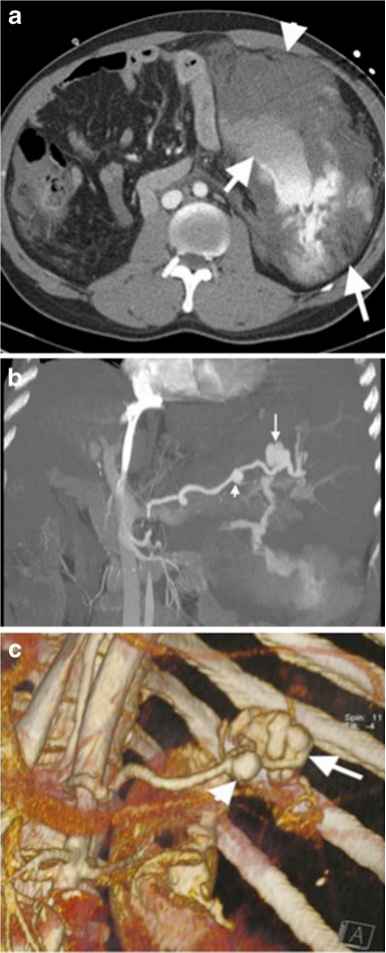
Ruptured splenic artery aneurysm. (a) Axial contrast-enhanced CT of a 57-year-old man with shock findings demonstrates a splenic artery aneurysm (*short arrow*) with surrounding active haemorrhage (*arrowhead*). Spleen (*long arrow*) has an heterogeneous appearance secondary to perfusion deficiency resulting from aneurysm haemorrhage. (b) Coronal MIP and (c) VR CT images reveal a large (*arrows*) and small (*arrowheads*) aneurysms
